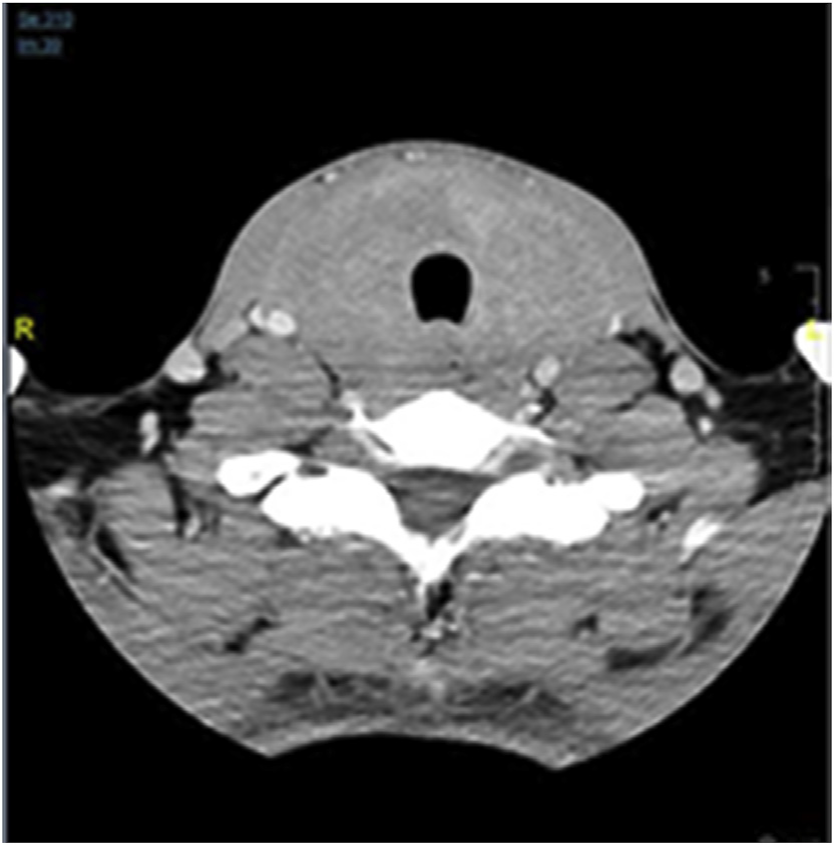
CT-Scan neck showed diffusely enlarged thyroid gland with heterogeneous enhancement, no calcification and minimal retrosternal extension. There was no tracheal compression or displacement but both common carotids and internal jugular veins were displaced posterolaterally. Multiple small suspicious lymph glands were noted at level II, III, IV and paratracheal region bilaterally.
